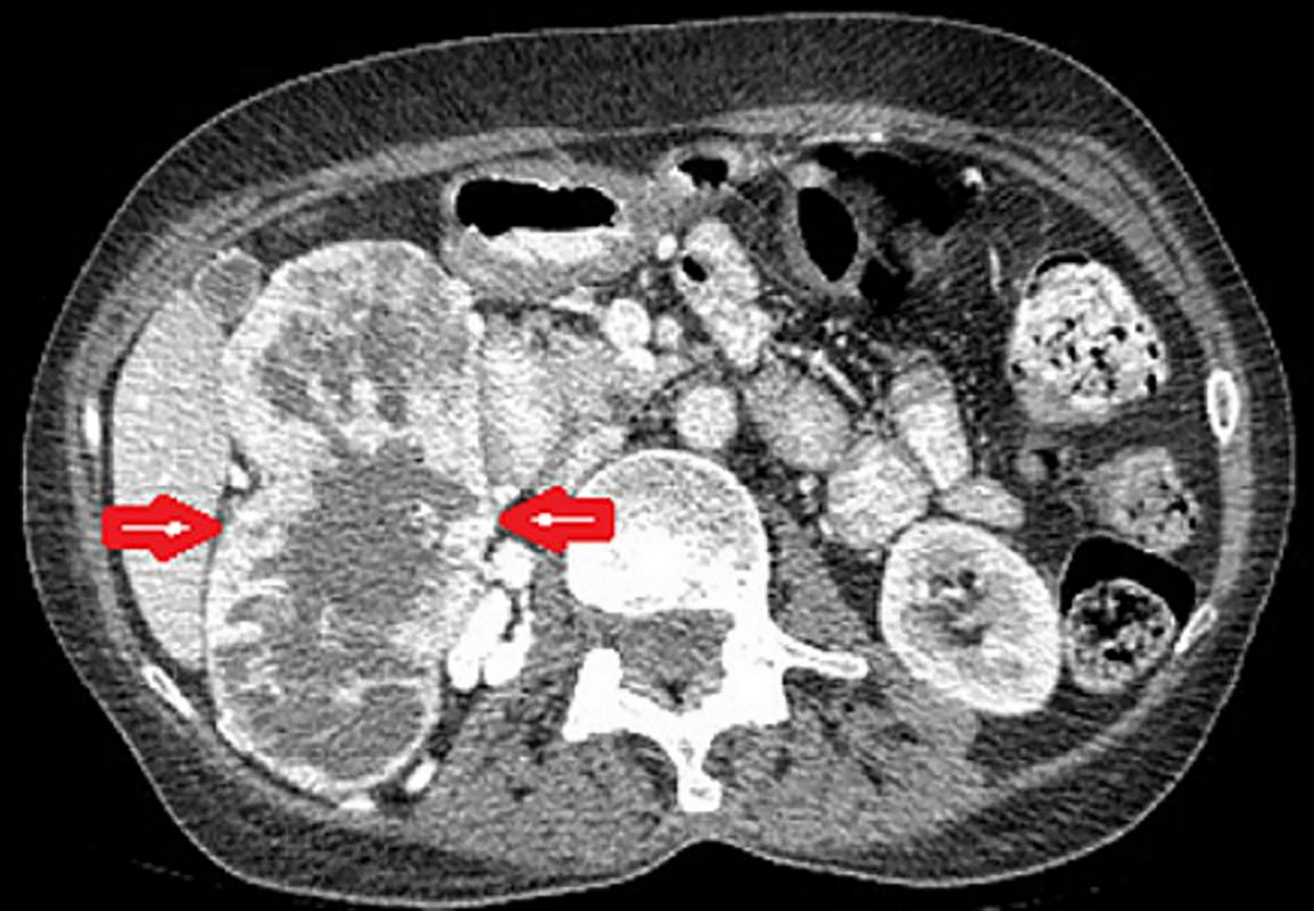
Abdominopelvic CT scan with intravenous contrast injection reveals right renal mass with heterogeneous enhancement.
